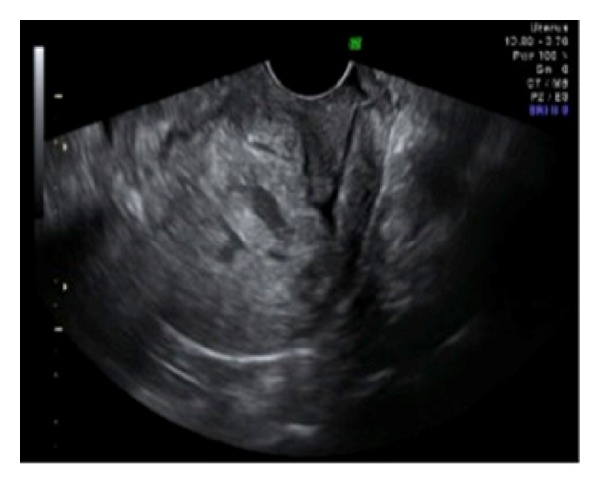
Ultrasonography showing soft tissue density with irregular contour in the endometrial cavity.
